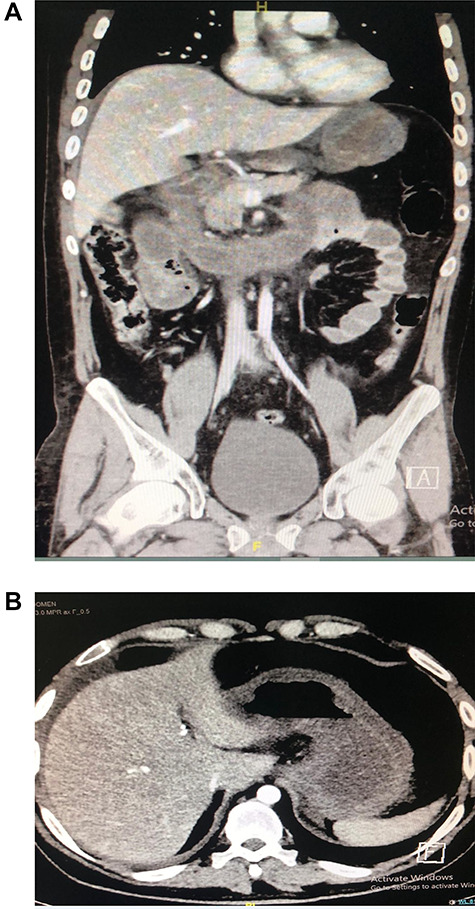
(A and B). CT showing segmental dilatation of the small bowel with diffuse circumferential wall thickening and suspected tow areas of transnational zones.
